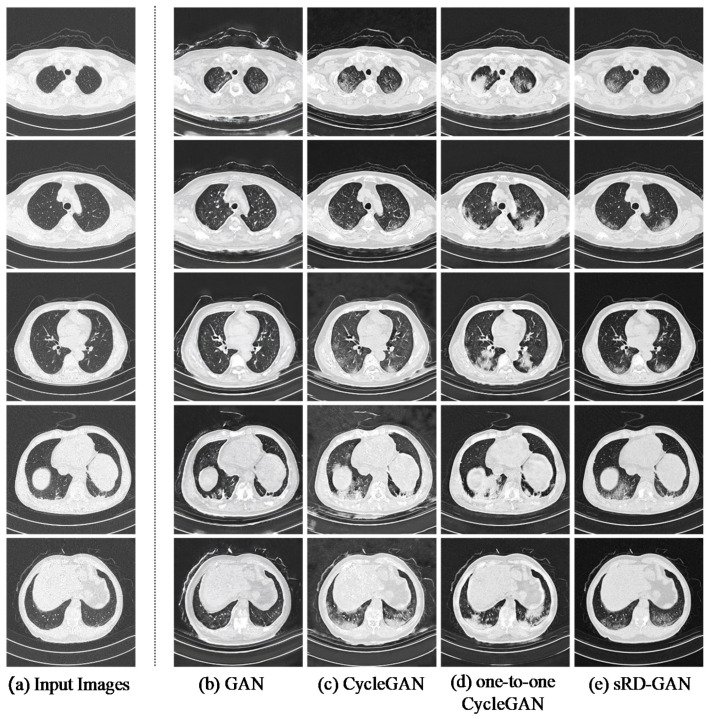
(a) Real COVID-19 CT images, (b–e) example of synthetic images generated by the proposed sRD-GAN and other GAN baselines. The synthetic images generated from GAN and CycleGAN contain a suboptimal realistic representation of the synthetic instances with large magnitude of noise artifacts.
